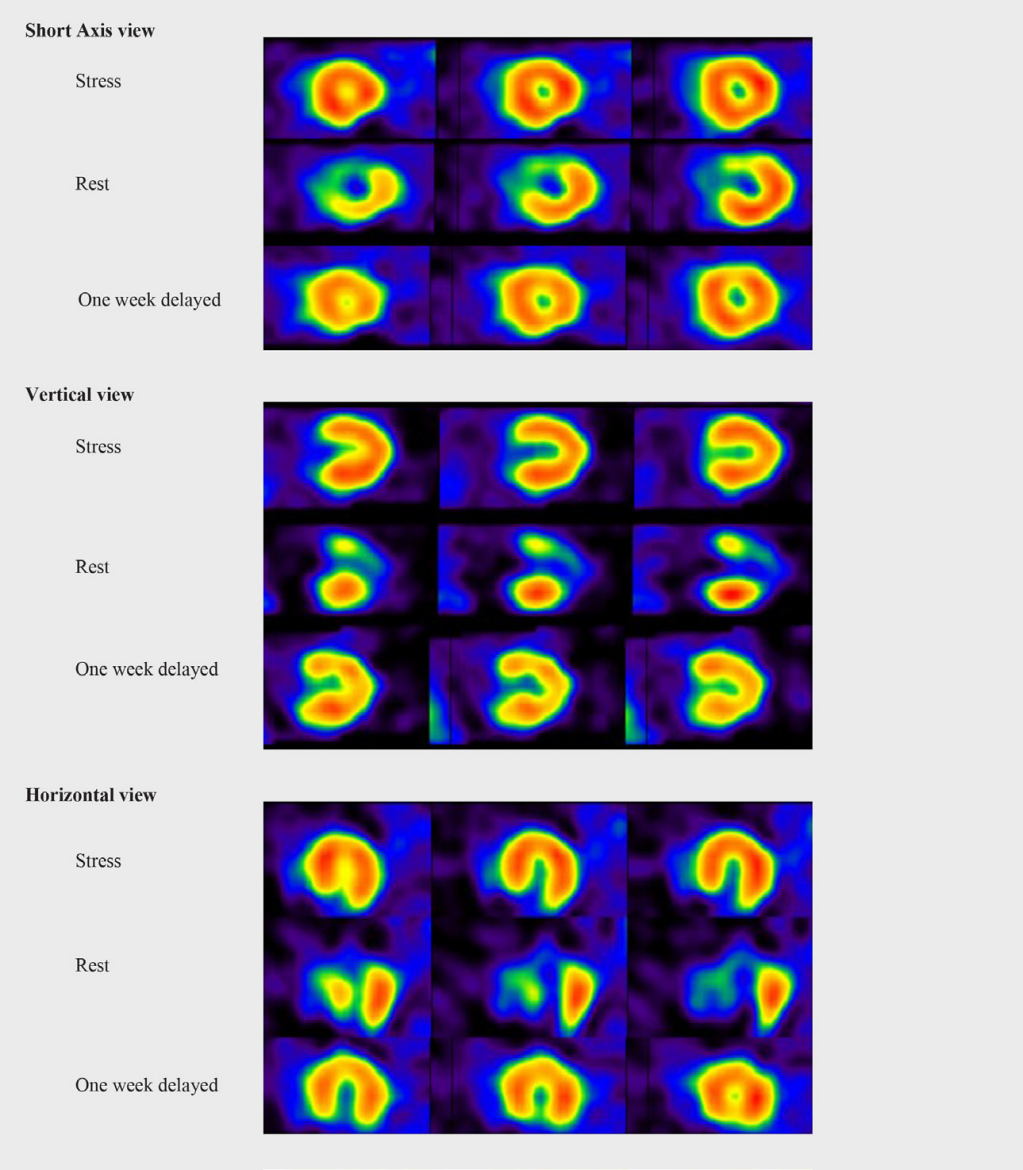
Myocardial perfusion single-photon emission computed tomography of a 52-year-old patient with temporary at-rest perfusion defects (Takotsubo syndrome); normal stress (dipyridamole), perfusion defect at the anterior, anteroseptal, and apical myocardial walls at rest (^99m^Tc-MIBI) and normal 1 week’s delayed repeat rest images (Tl-201).
